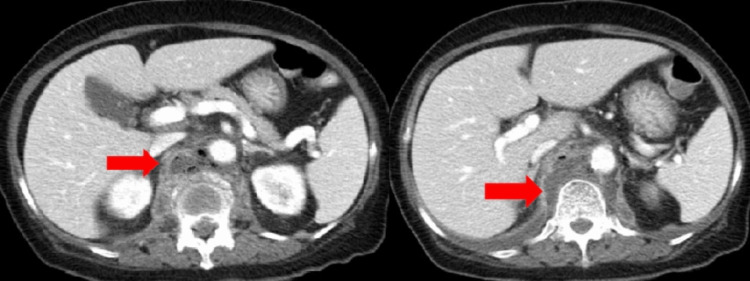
Abdominal and pelvic computed tomography axial view (L1 level): abscess pocket (red arrows).
